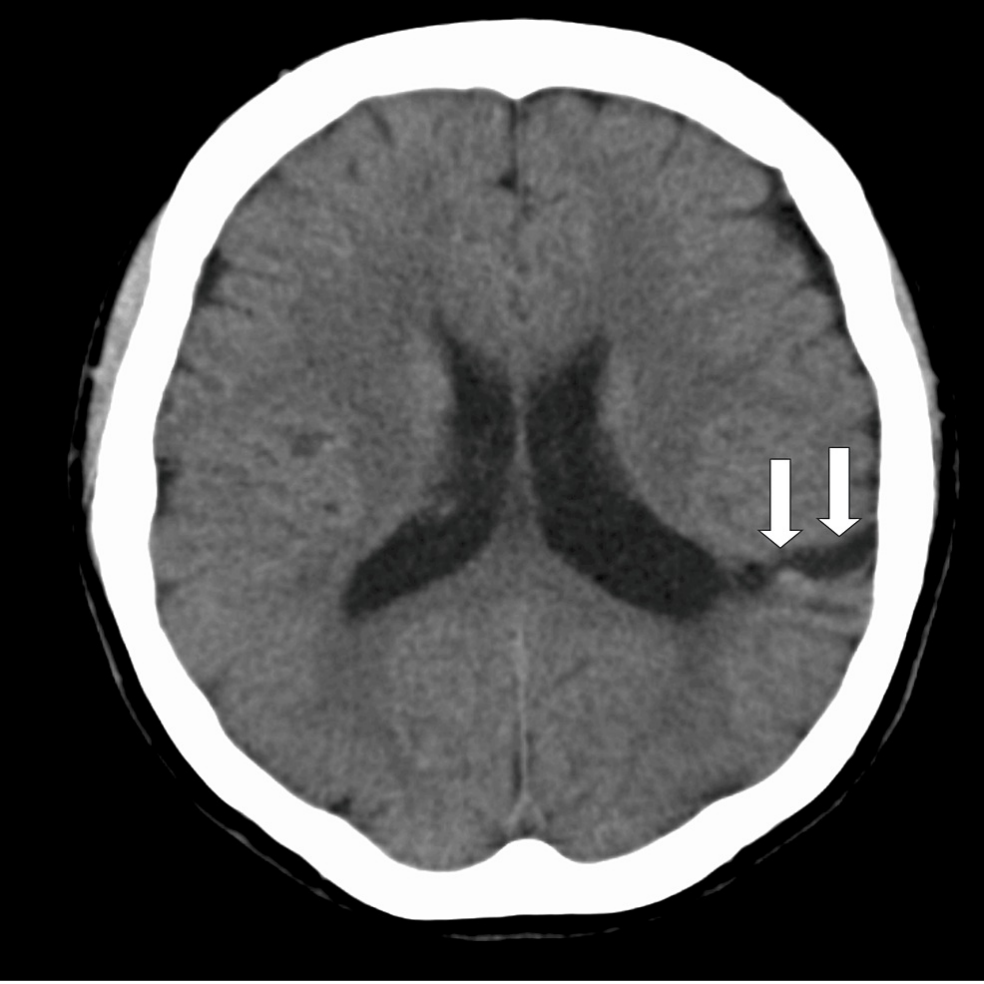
Head CT image Head computed tomography revealed a hypo-absorptive zone in the left temporal lobe (shown by white arrows), which is considered to be a finding of cerebral hemorrhage.
